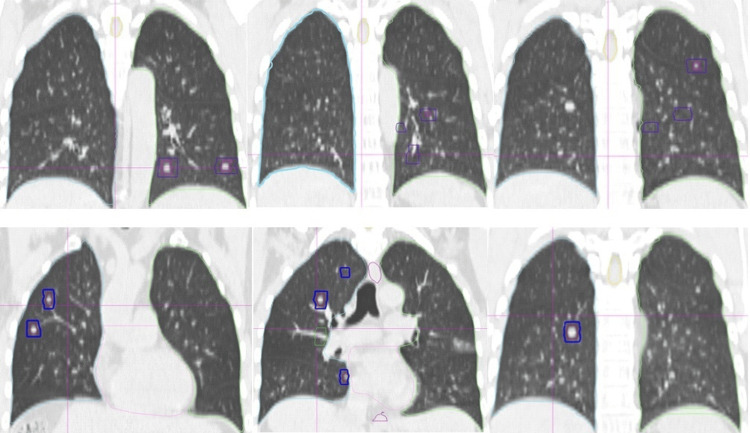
Target delineation of total lung carbon ion radiotherapy (CIRT) of Case 1 presented in coronal CT images with the lung window. Because the lesions were spread three-dimensionally, not all the 16 targets are visible in the figures. The targets of the left lung (upper panel) and right lung (lower panel) are shown separately. Red: gross target volume (GTV); blue: planning target volume (PTV).
